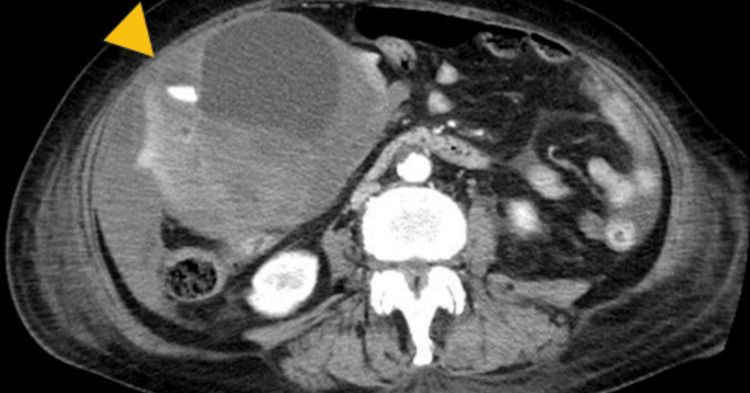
Contrast-enhanced computed tomography scan showing intratumoral extravasation (arrowhead) during the arterial phase and hemoperitoneum due to rupture of the tumor.
